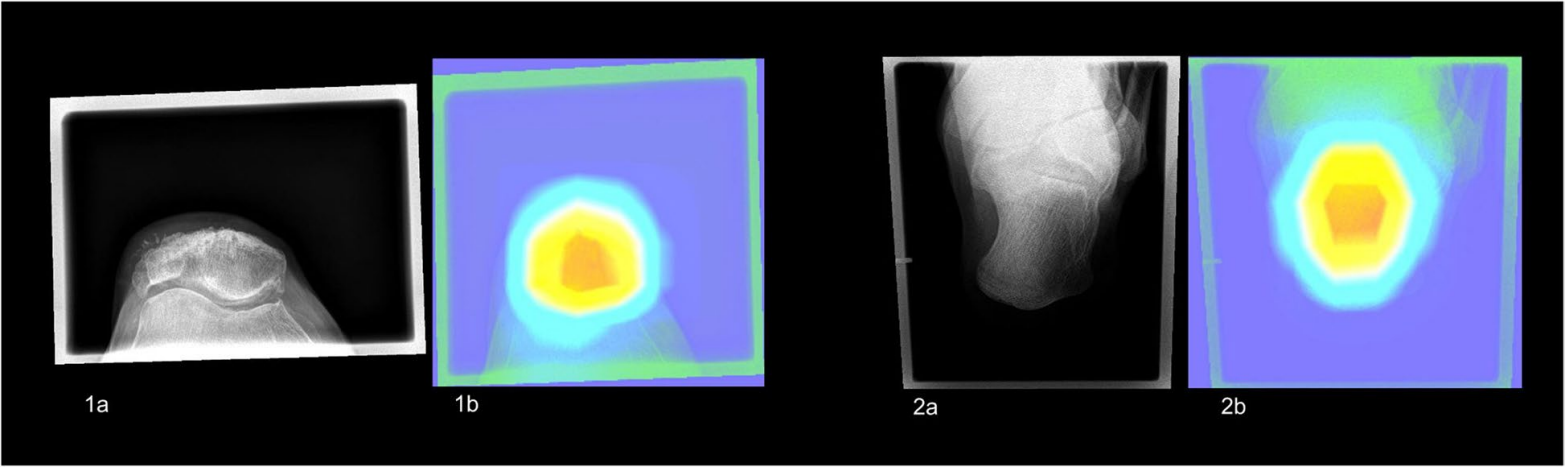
Input radiographs resized to 256 × 256 pixels with corresponding Grad-CAM overlay of two correctly classified radiographs for the body side demonstrating the influential image regions (red overlay). 1a, 1b Right patella. 2a, 2b Left calcaneus
